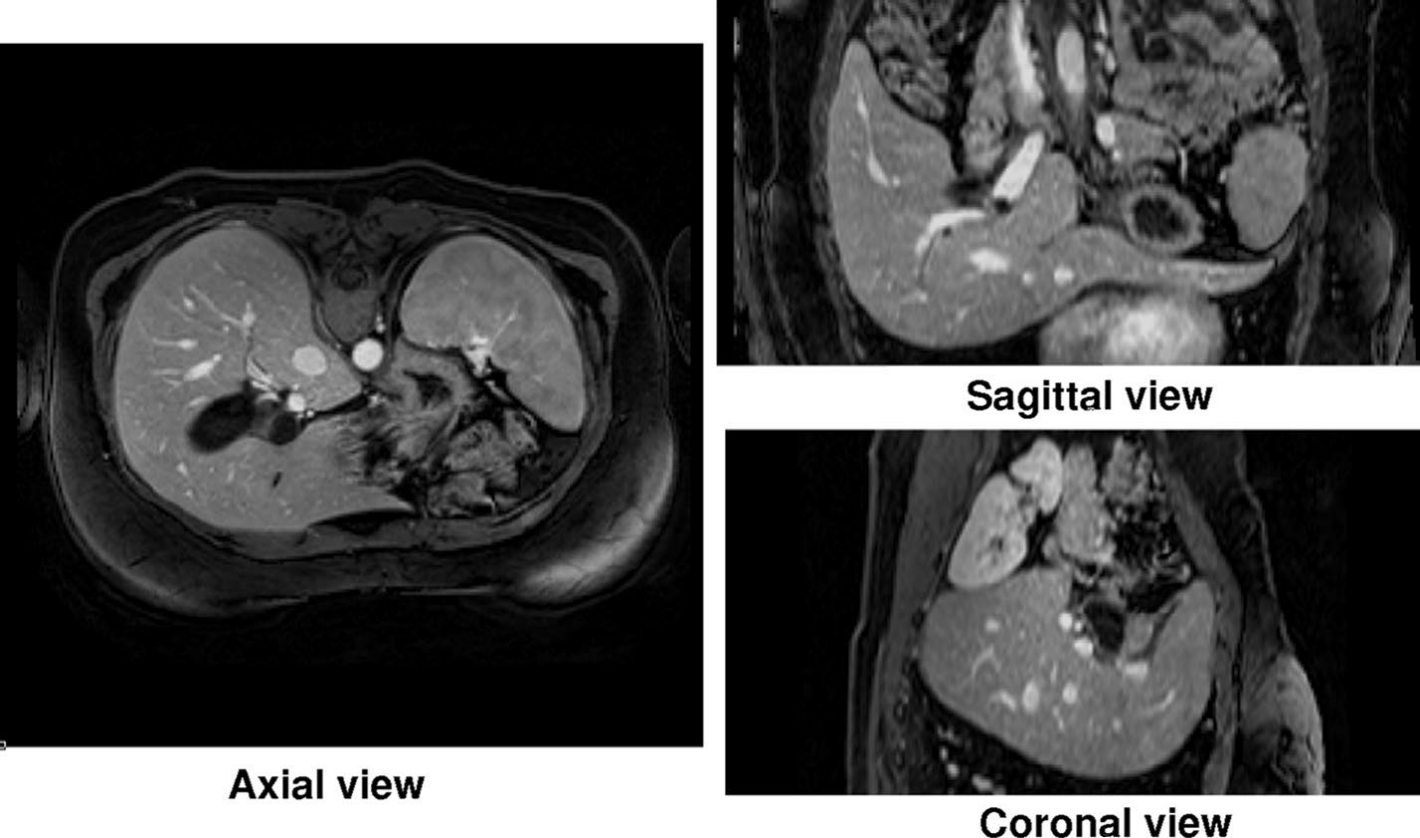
Example of original images, as captured by the MRI scanner
